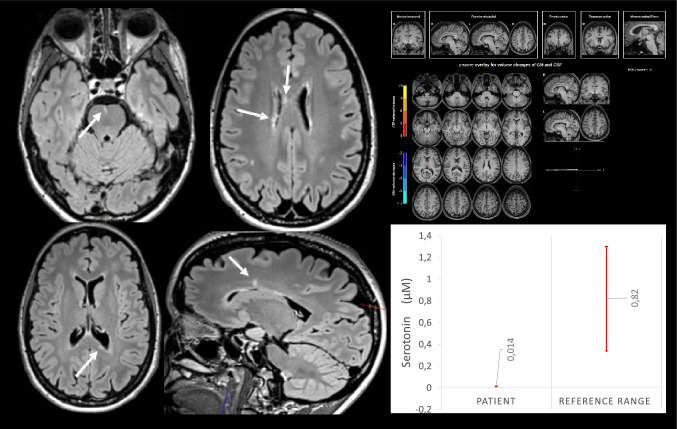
White matter magnetic resonance imaging lesions are marked with arrows. The automated magnetic resonance imaging analysis (https://www.veobrain.com/?page=veomorph) did not detect any atrophic changes. The low serotonin levels in cerebrospinal fluid are shown in comparison to known reference values from controls (Mandal et al. 2012). *CSF* cerebrospinal fluid, *GM* grey matter, *L* left, *R* right
